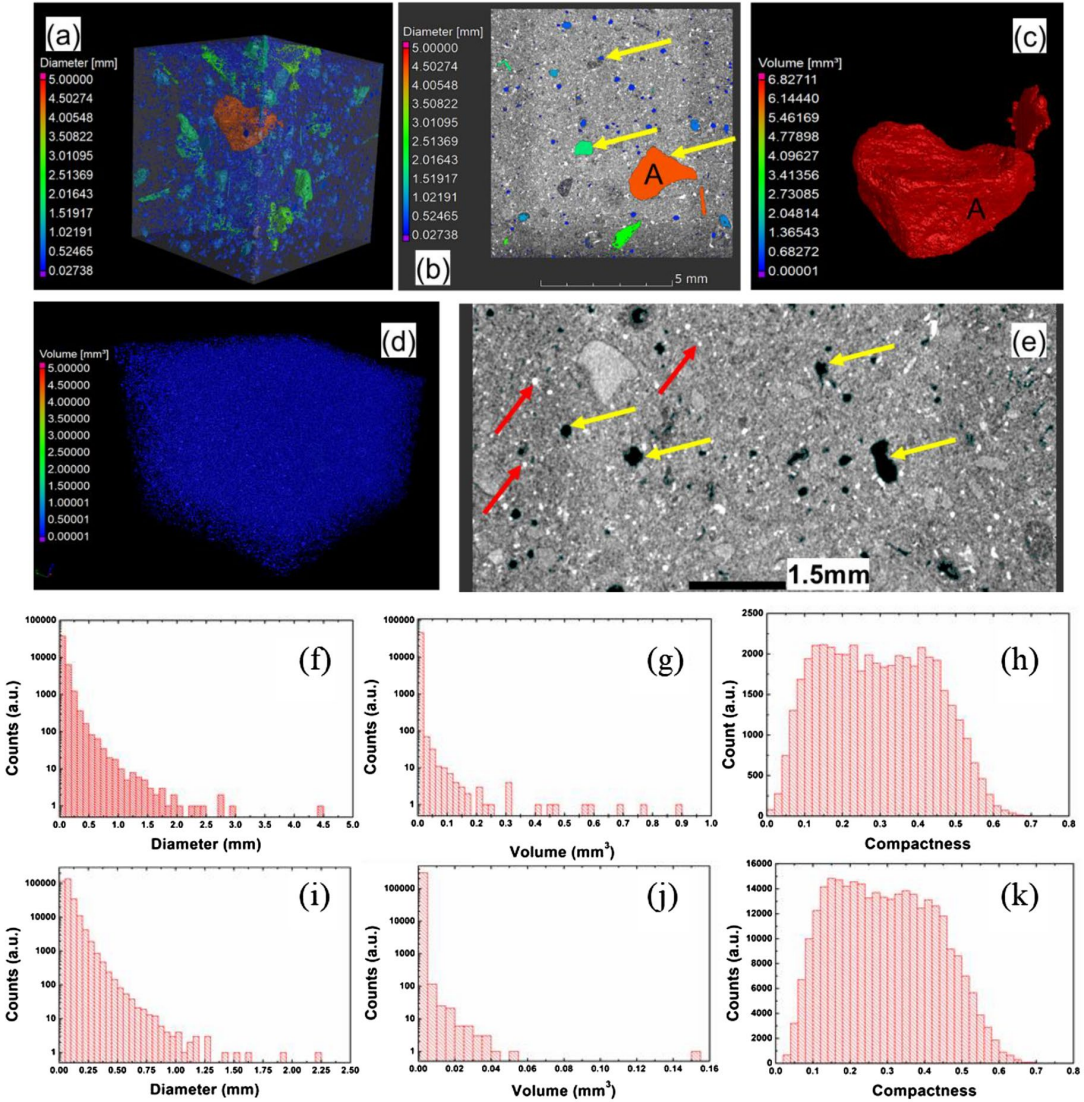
X-ray tomography on a sample from the burnt brick (B): (a) 3D rendering showing the pores; (b,e) 2D sections; (c) 3D rendering of the largest pore identified in the sample; (d) white particles mainly ascribed to quartz phase (see text); (f–h) diameter, volume and compactness distributions of the pores in the investigated sample; (i–k) diameter, volume and compactness distributions of the white particles in the investigated sample. Yellow arrows indicate the pores and red ones the white particles.
